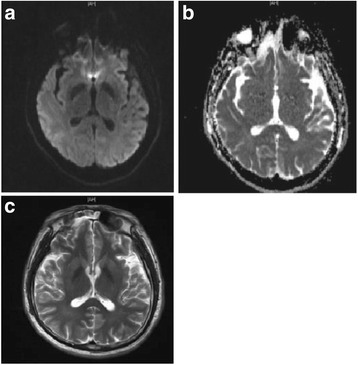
Follow up cranial MRI. The follow up cranial MRI showed no lesion on any sequence (﻿﻿a: DWI, b:﻿ ADC, c: T2WI)
